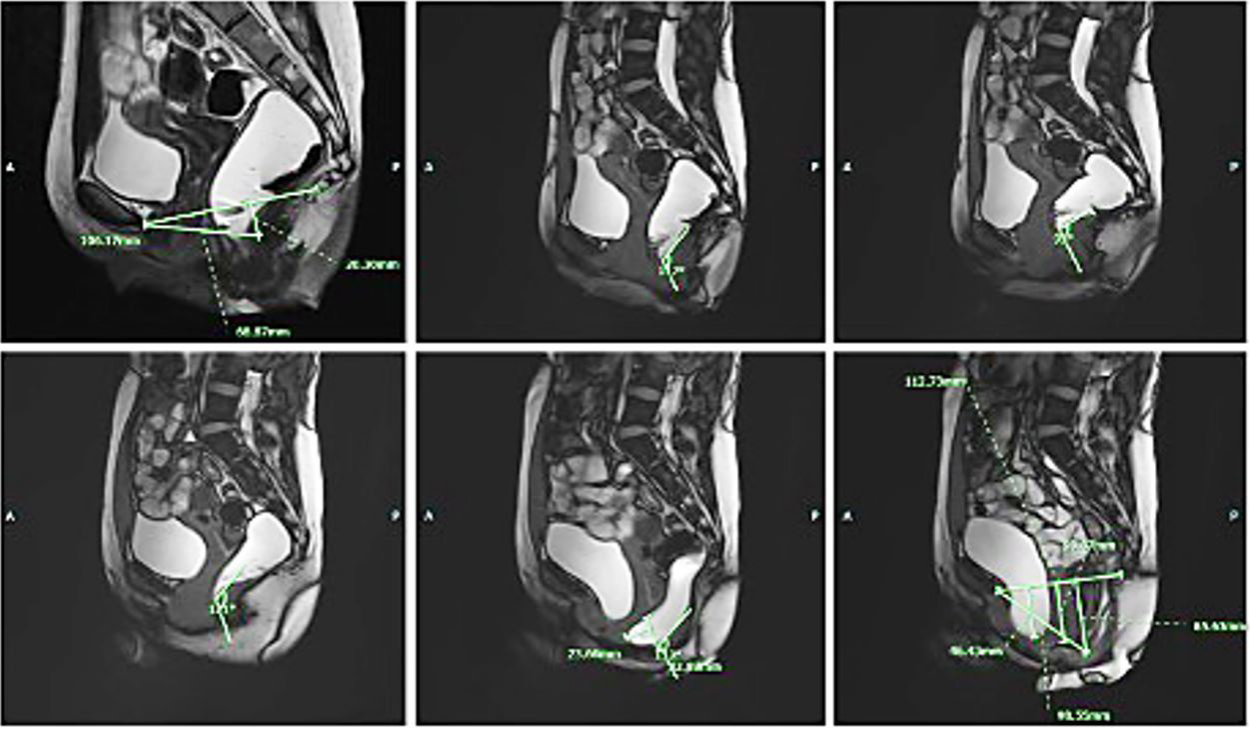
MRI defecography. Findings were suggestive of tricompartmental pelvic floor prolapse, moderate size anterior rectocele, and grade 2 sigmoidocele.
